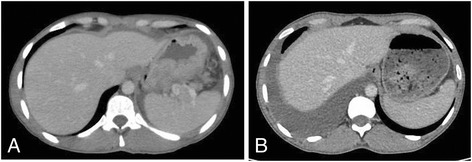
Abdominal CT showing gastric wall before (a) and after (b) the BISIC regimen. A significant response with regression of gastric wall thickening can be observed
